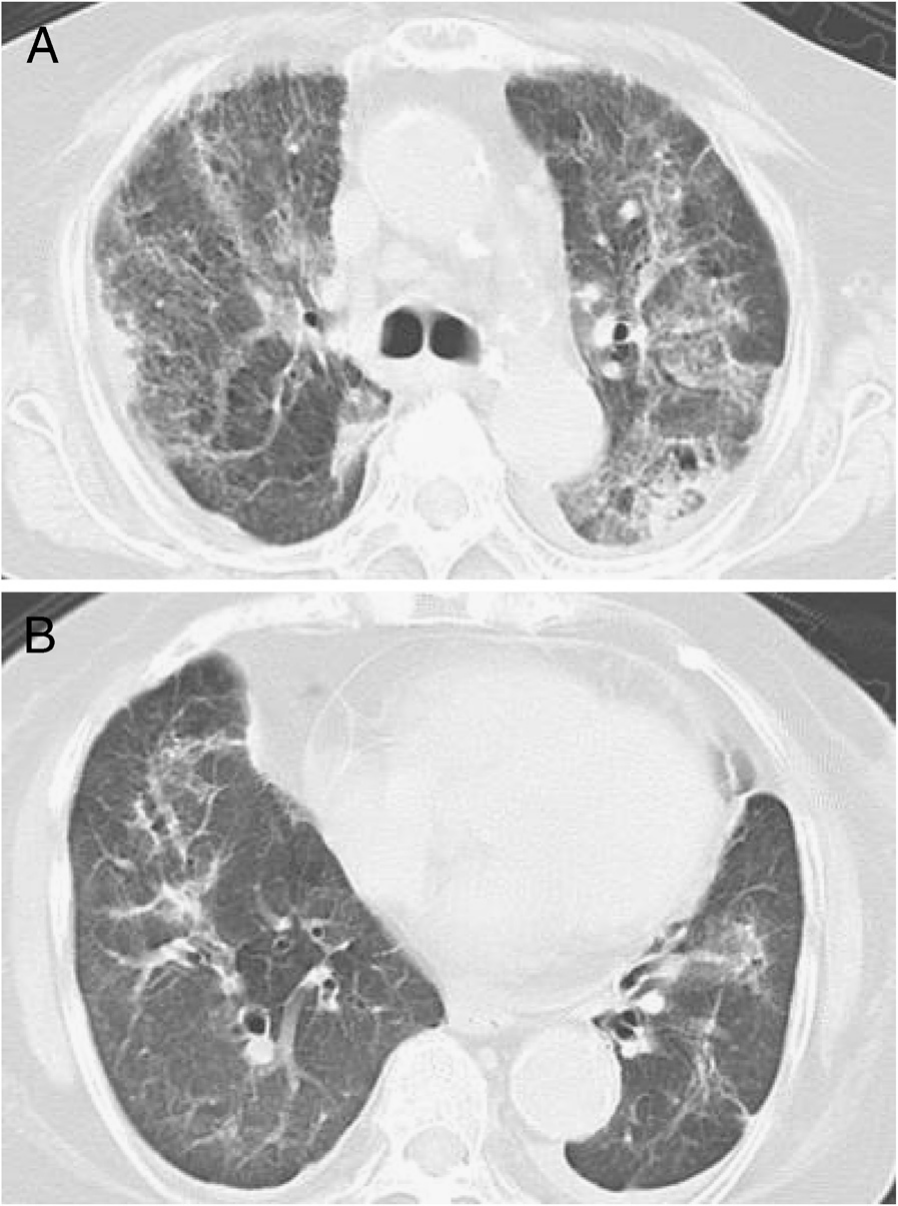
A chest computed tomography scan obtained after the diagnosis of pneumocystis pneumonia (upper lung field: 3A, lower lung field: 3B) showed areas of nonsegmental ground-glass opacity and subpleural curvilinear shadows bilaterally in all lobes.
